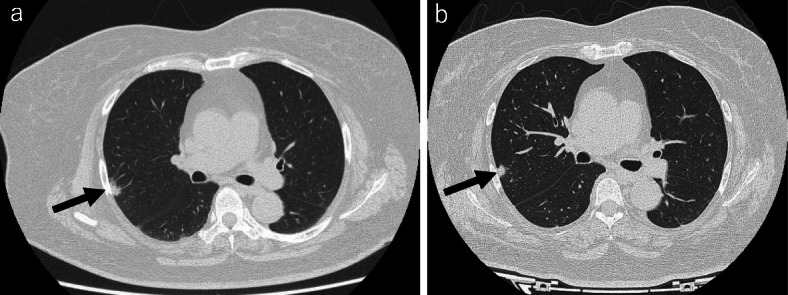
Chest computed tomography scan. a An image obtained 1 month before the LSG is shown. A 10-mm mass was observed in the superior lobe of the right lung (arrow). b An image obtained 2.5 months after the LSG is shown. No marked change in the size of the lung mass (arrow) was noted, but a reduction in the amount of subcutaneous fat was seen
